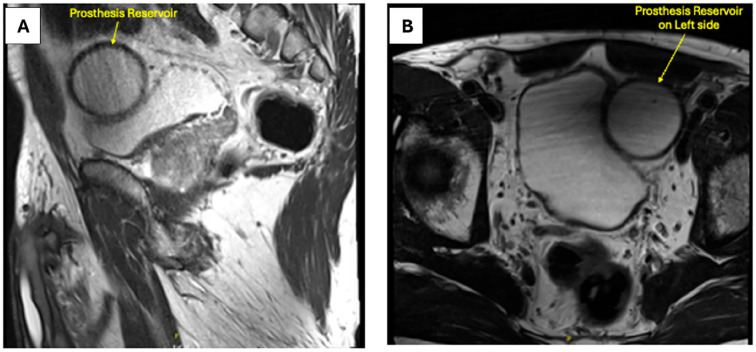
Representative preoperative pelvic magnetic resonance imaging (MRI) demonstrating the presence and location of a pre-existing penile prosthesis reservoir in patients undergoing robot-assisted radical prostatectomy.
